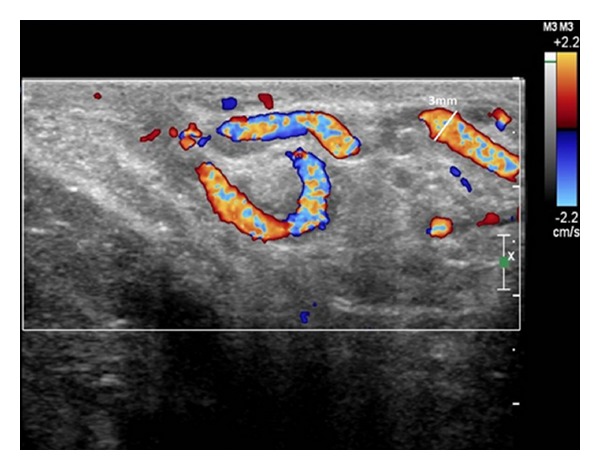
Right testicle doppler ultrasound, transverse superior view, showing right-sided varicocele with mild dilatation (3 mm) of vessels of the pampiniform plexus. There was no corresponding dilatation of vessels of the left pampiniform plexus.
